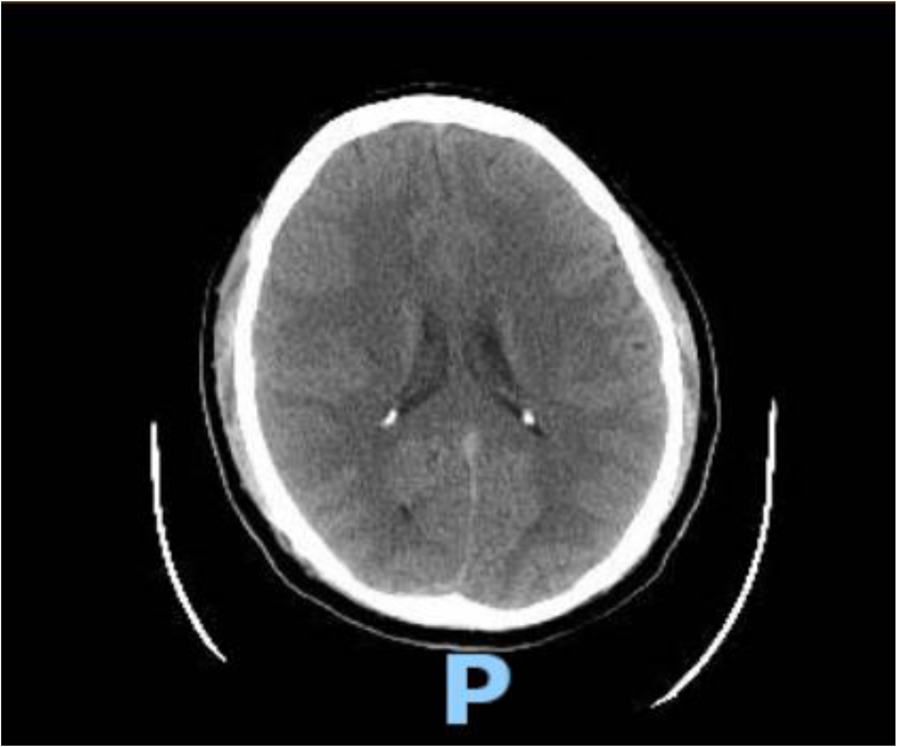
CT head with unremarkable findings (Axial plane)
